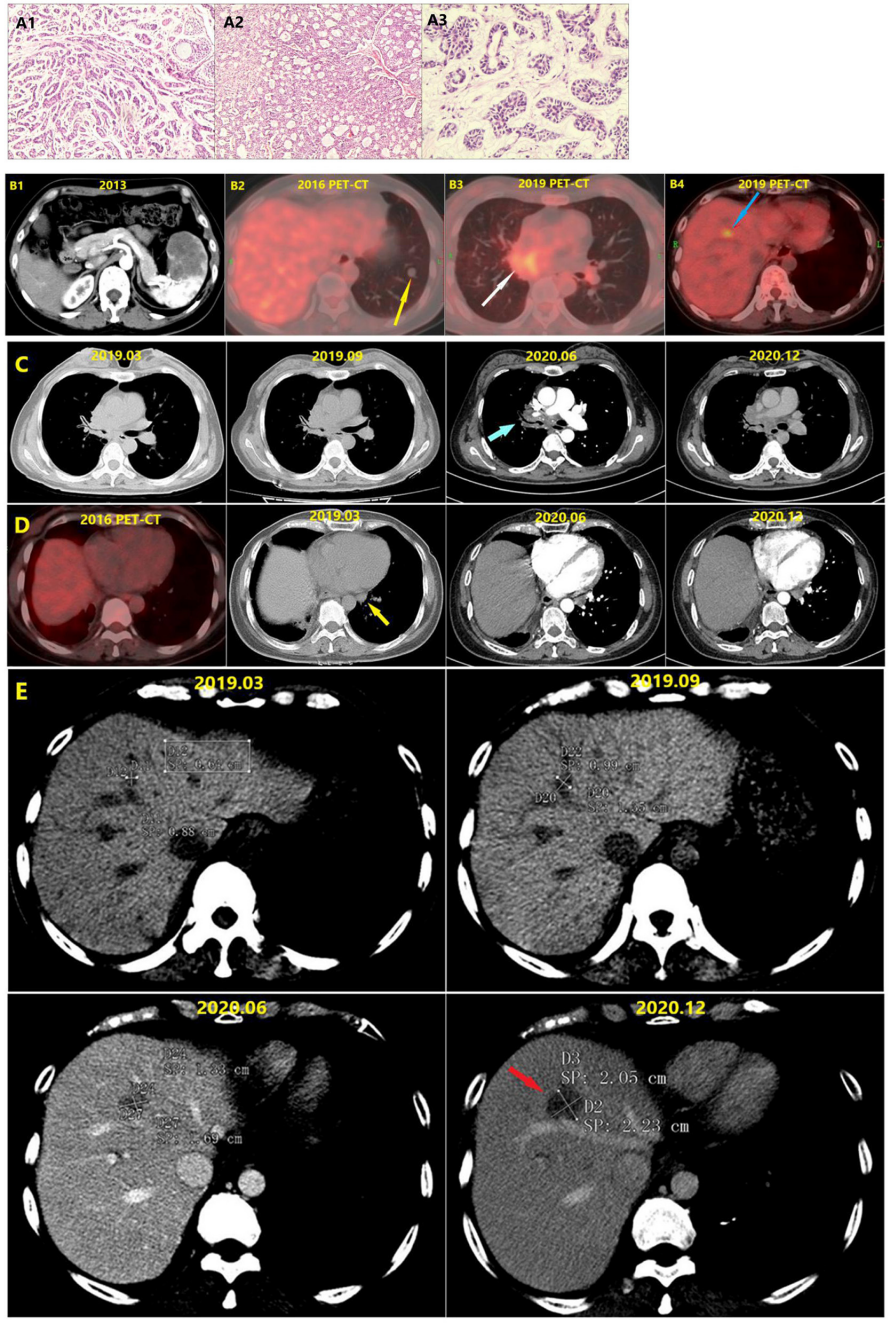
(A1) Pathological section of adenoid cystic carcinoma of the right middle lung. (A2) Pathological section of the spleen metastasis. (A3) Pathological section of the left lower lung metastasis. (B1) The spleen metastasis. (B2) The left lower lung metastasis (Yellow arrow). (B3) The PET-CT in December 2019 showed tumor recurrence in the right hilus pulmonis (White arrow). The bronchoscope finds adenoid cystic carcinoma cells at the end of the right main bronchus. (B4) The liver metastasis (Blue arrow). (C) Changes of the lesions at hilum of right lung (Blue arrow) in different periods. Comparison of the conditions 9 months before and 6 months after the targeted therapy showed that no significant change occurred to the lesions. (D) Changes of the left hilar lymph node in different periods. In 2016, PET-CT showed no lymph node at the hilum of left lung. Comparison of the conditions 9 months before and 6 months after the targeted therapy showed that no significant change occurred to the size of left hilar lymph node (Yellow arrow). (E) Changes of liver metastasis (Red arrow) in different periods.
